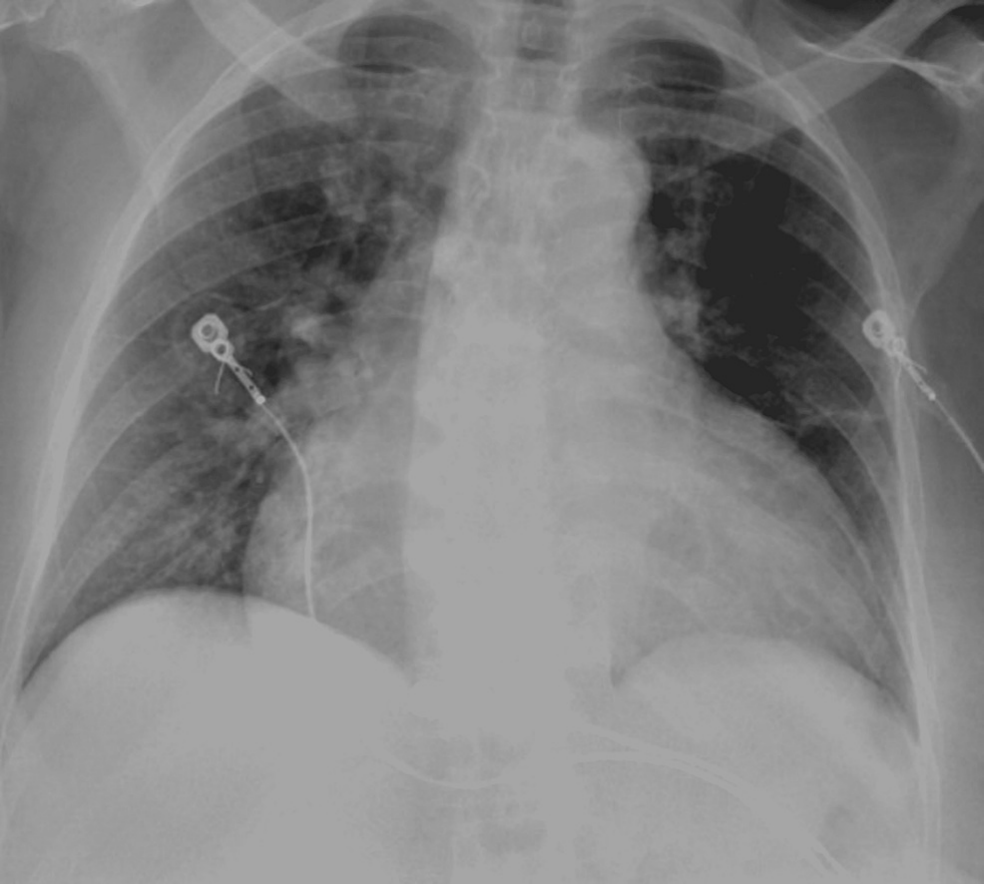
Chest X-ray exhibiting cardiomegaly with mild congestive failure pattern.
